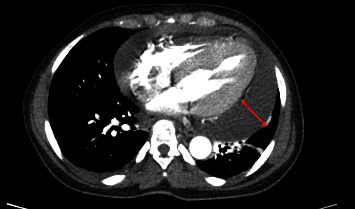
Chest CT with angiography: axial view at midthoracic level shows large pericardial effusion (red line) without pericardial or pleural thickening, or pericardial or pleural masses.
